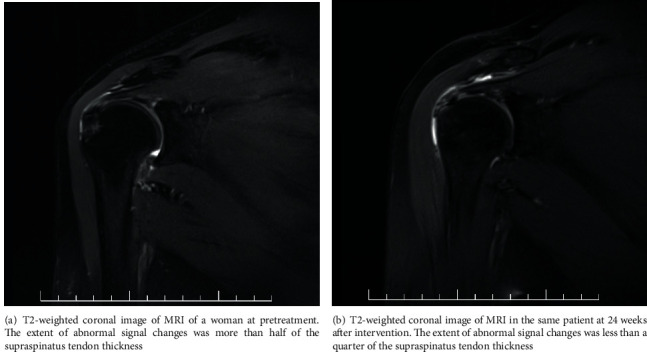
MRI findings of supraspinatus tendinopathy.
